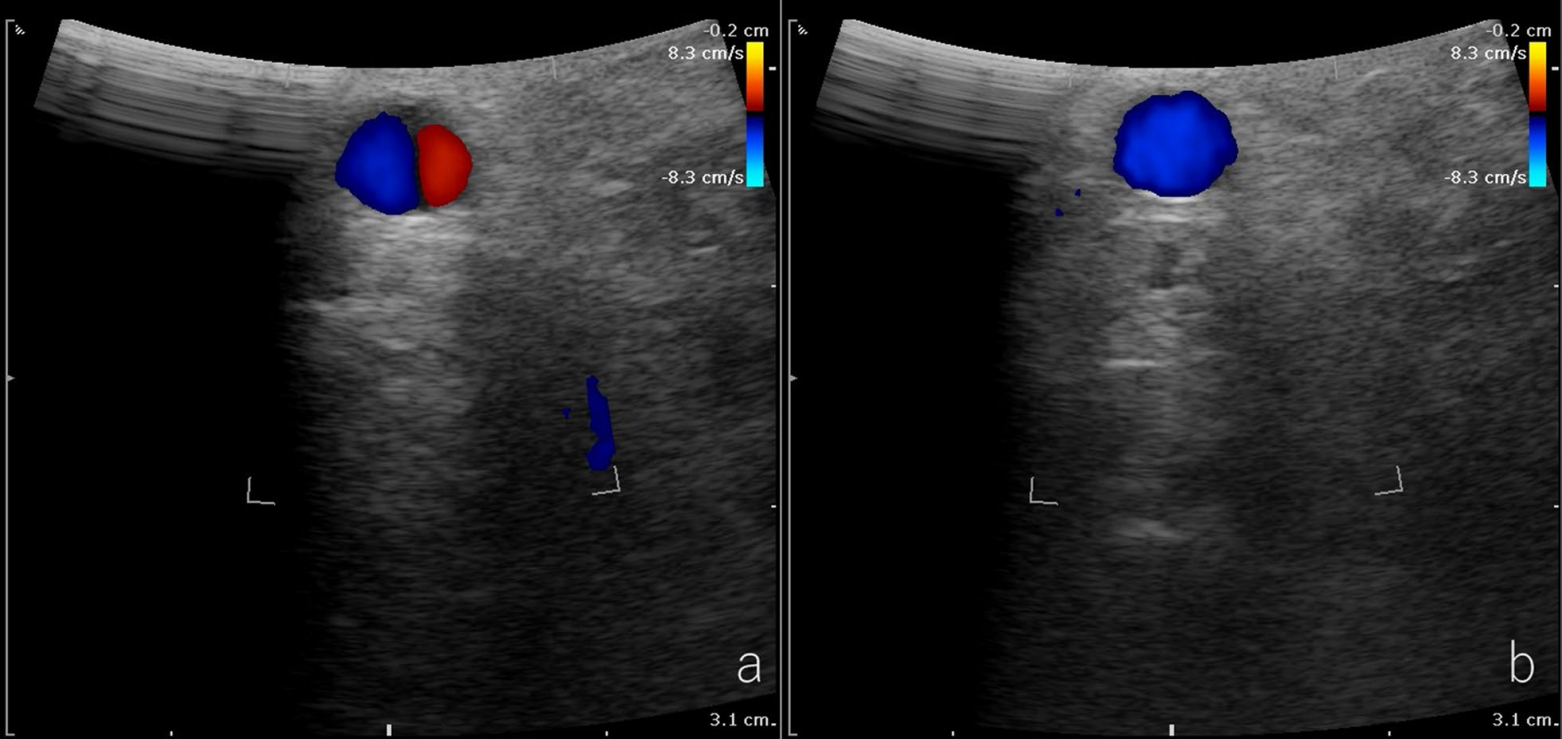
Intraoperative ultrasonography of CHA. a: Before sectioning the median arcuate ligament, retrograde blood flow in the common hepatic artery (CHA) is observed. b After releasing the compression, antegrade blood flow in the CHA is depicted
